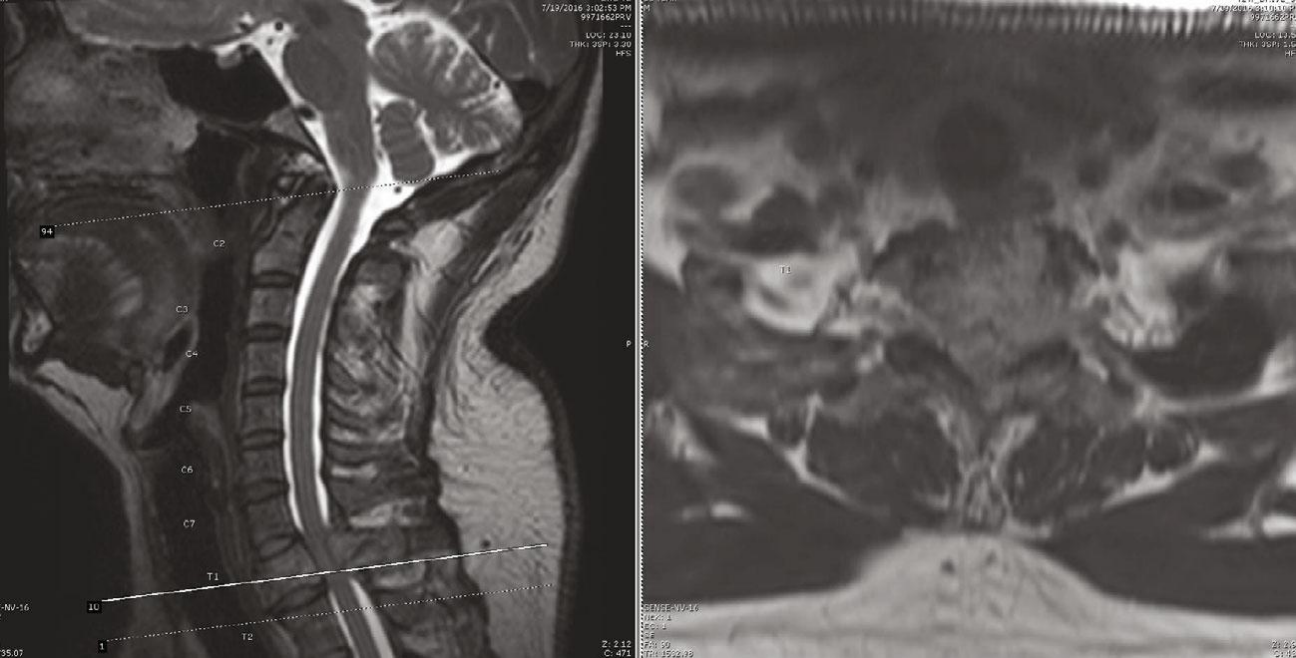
Preoperative T2 magnetic resonance imaging showing sagittal and axial views.
